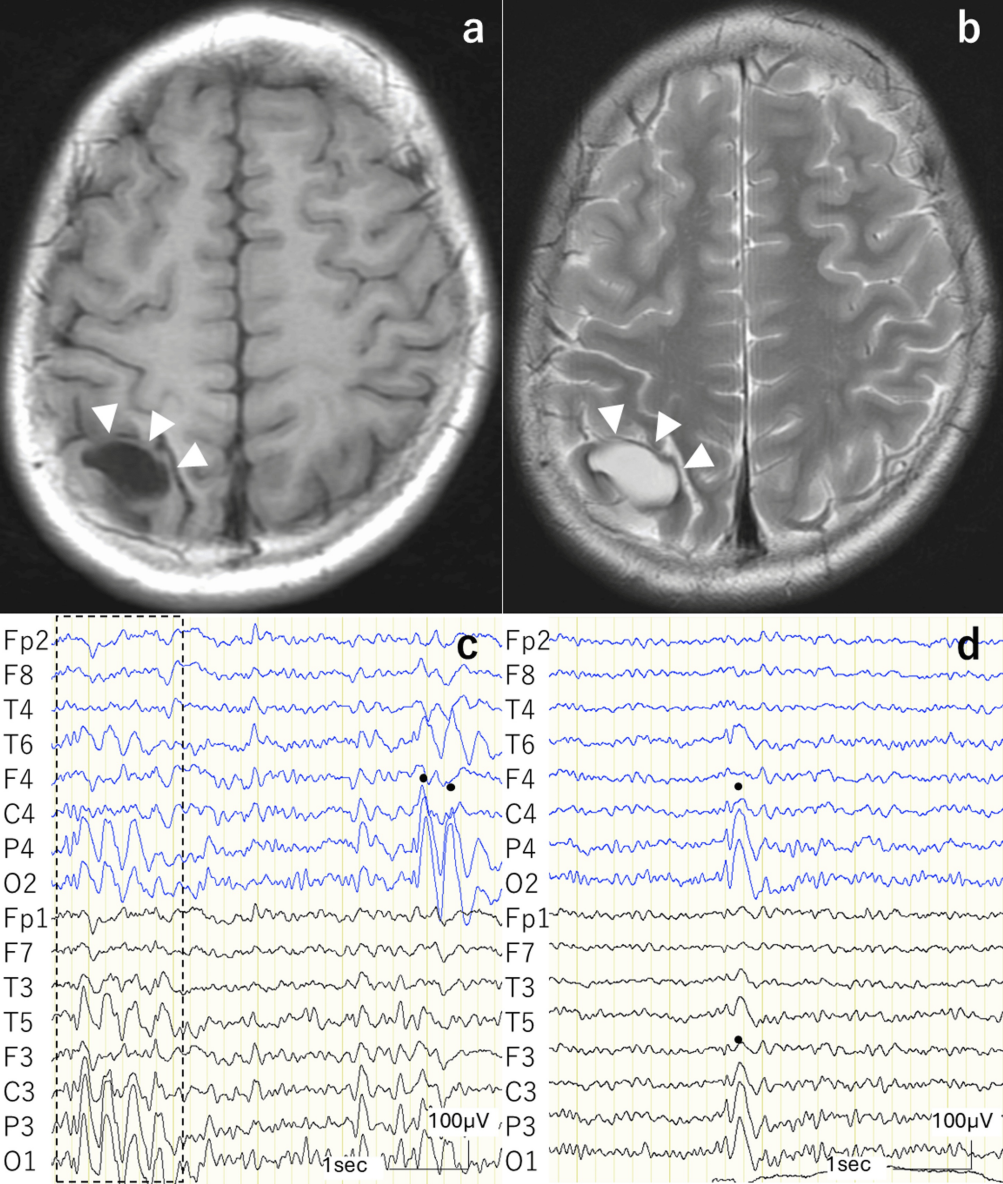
MRI and EEG findings of Case 2 (a) T1 and (b) T2-weighted images show a cystic lesion in the right parietal lobe (arrowhead). The lesion was surrounded by T2 low signal intensity, suggesting hemosiderin deposition. (c) Referential montage EEG in the acute phase showing bilateral parieto-occipital (surrounded by a dotted line) and right parieto-occipital (dot) spike-and-waves. (d) EEG performed three years after the convulsion showed bilateral parieto-occipital spike-and-waves (dots).
